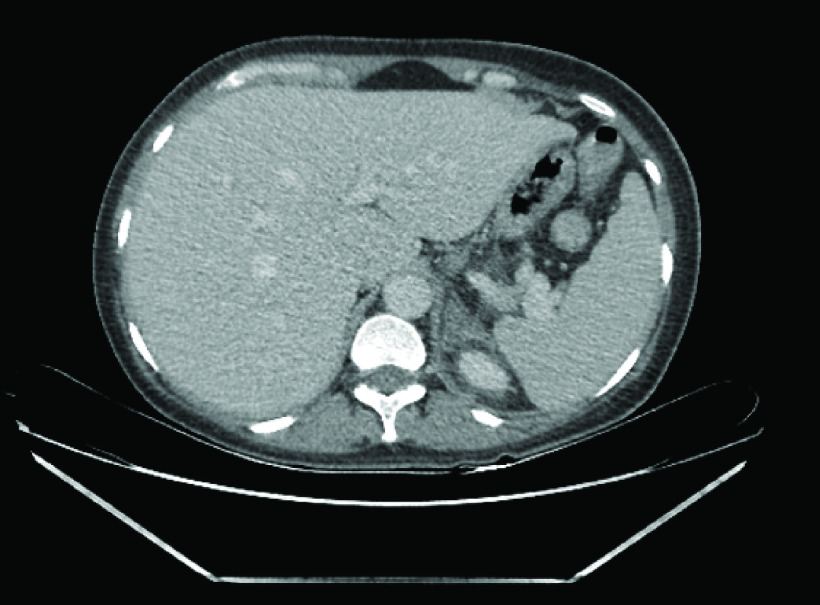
Computed tomography scan postpartum showing nonhemorragic adrenal infarction on the left side.
